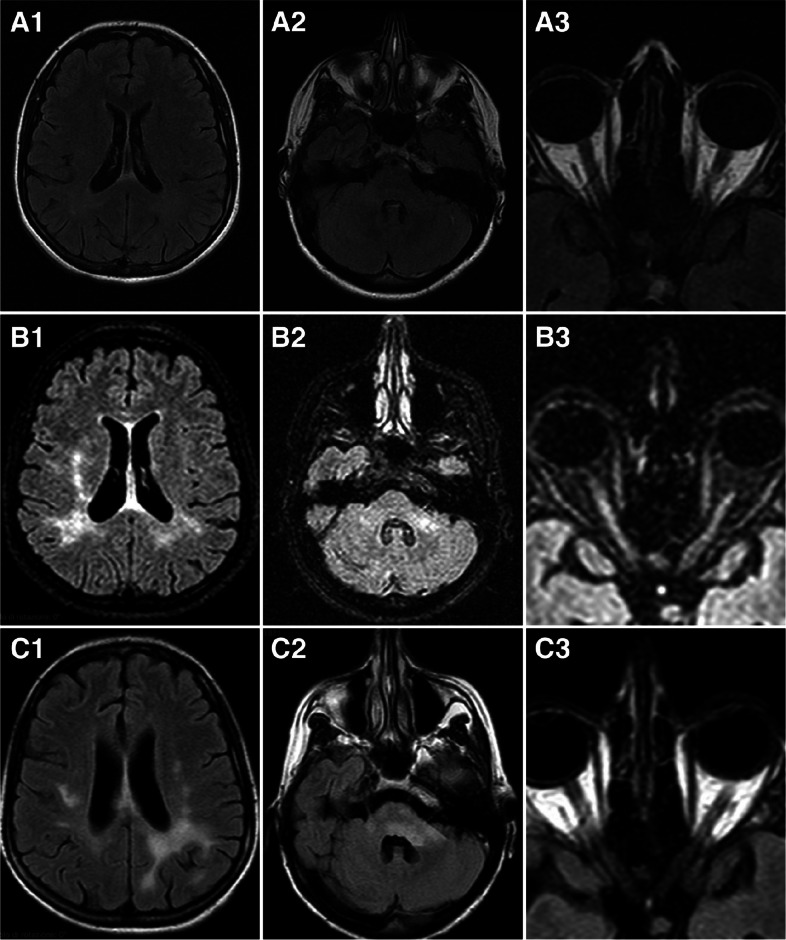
A MRI performed in 2012 showing the right temporo-occipital white matter hyperintensity in the axial T2-FLAIR WI (A1), the absence of lesion at infratentorial level documented in the axial T2-FLAIR-WI (A2) and the focal hyperintensity of the left optic nerve in the axial T2-FLAIR WI (A3). B MRI performed in 2017 showing the increasing of whiter matter hyperintensity involving the parieto-occipital lobes especially the right white matter in the axial T2-FLAIR WI (B1), the focal hyperintensity of the left middle cerebellar peduncle in the axial T2-FLAIR WI (B2) and the bilateral hyperintensity of optic nerves the axial T2-FLAIR WI (B3). C MRI performed in July 2018 showing the reduction of hyperintensity in right temporo-occipital white matter and the contemporary increase of hyperintensity on the left temporo-occipital white matter in the axial T2 FLAIR WI (C1), the increase of hyperintensity in the left middle cerebellar peduncle in the axial T2-FLAIR WI (C2) and the partial persistence of the hyperintensity in the bilateral optic nerves in the axial T2-FLAIR WI (C3)
